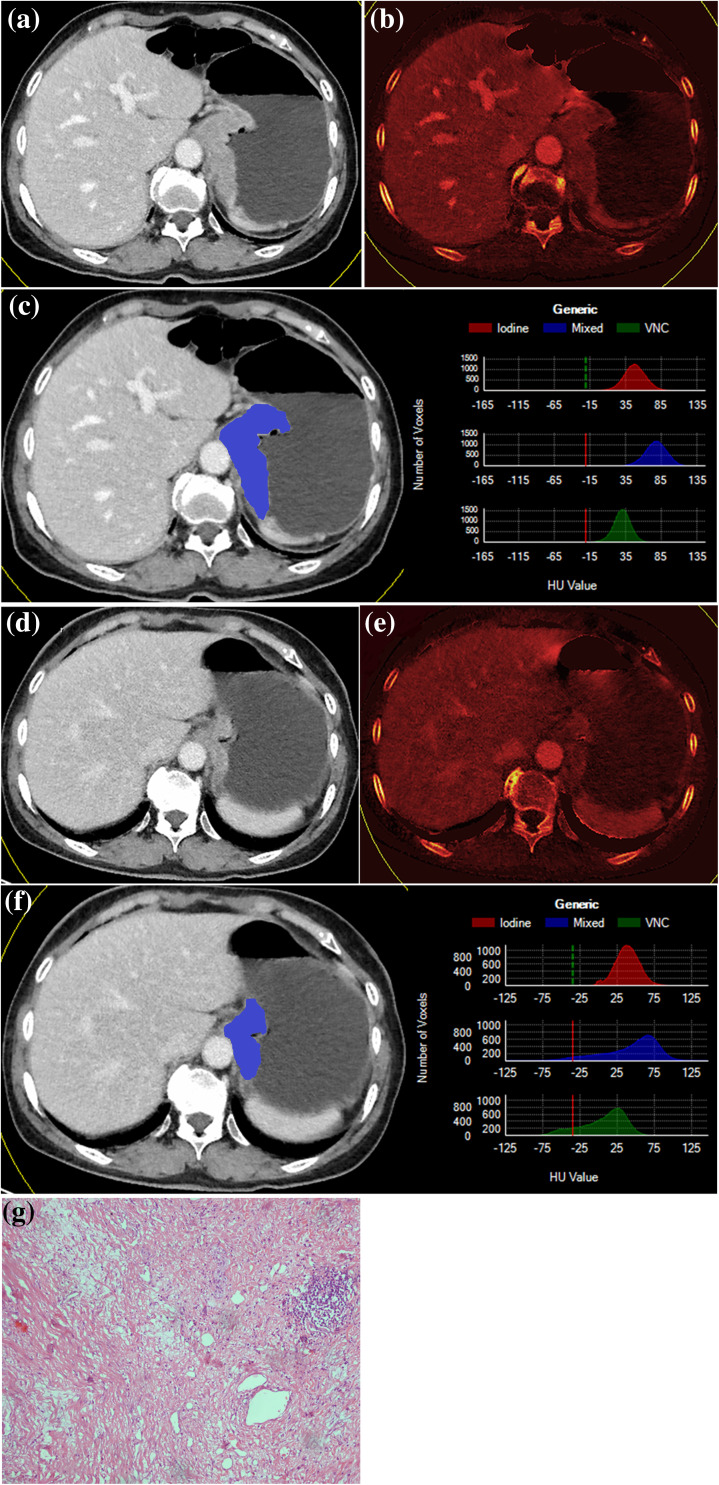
Baseline CT images (a–c) and follow-up CT images (d–f) of a patient (Female, 73 years) after three cycles of chemotherapy; a, d were mixed energy images of portal phase; b, e were corresponding iodine maps; right figures in c, f were the distribution of density values of the identified lesion in the iodine maps, mixed and virtual non-contrasted (VNC) images. The target lesion was semiautomatic segmented in 3D and with color-labeled tumor displayed on a representative axial slice, as shown in left in c and f (blue parts in tumor), (%Δ*S*: − 43.49%, %Δ*D*: − 15.92%, %ΔHU: − 15.88%, %Δ*V*: − 76.13%, %ΔTIU-p: − 78.93% for this patient). According to the WHO, RECIST criteria, this patient was classified as a non-responder, but according to other criteria, he was classified as a responder. The pathology of this patient (g) was grade 1, complete (0% residual tumor) tumor regression. Based on the definite diagnosis, this patient had survived 36 months and survives to this day
